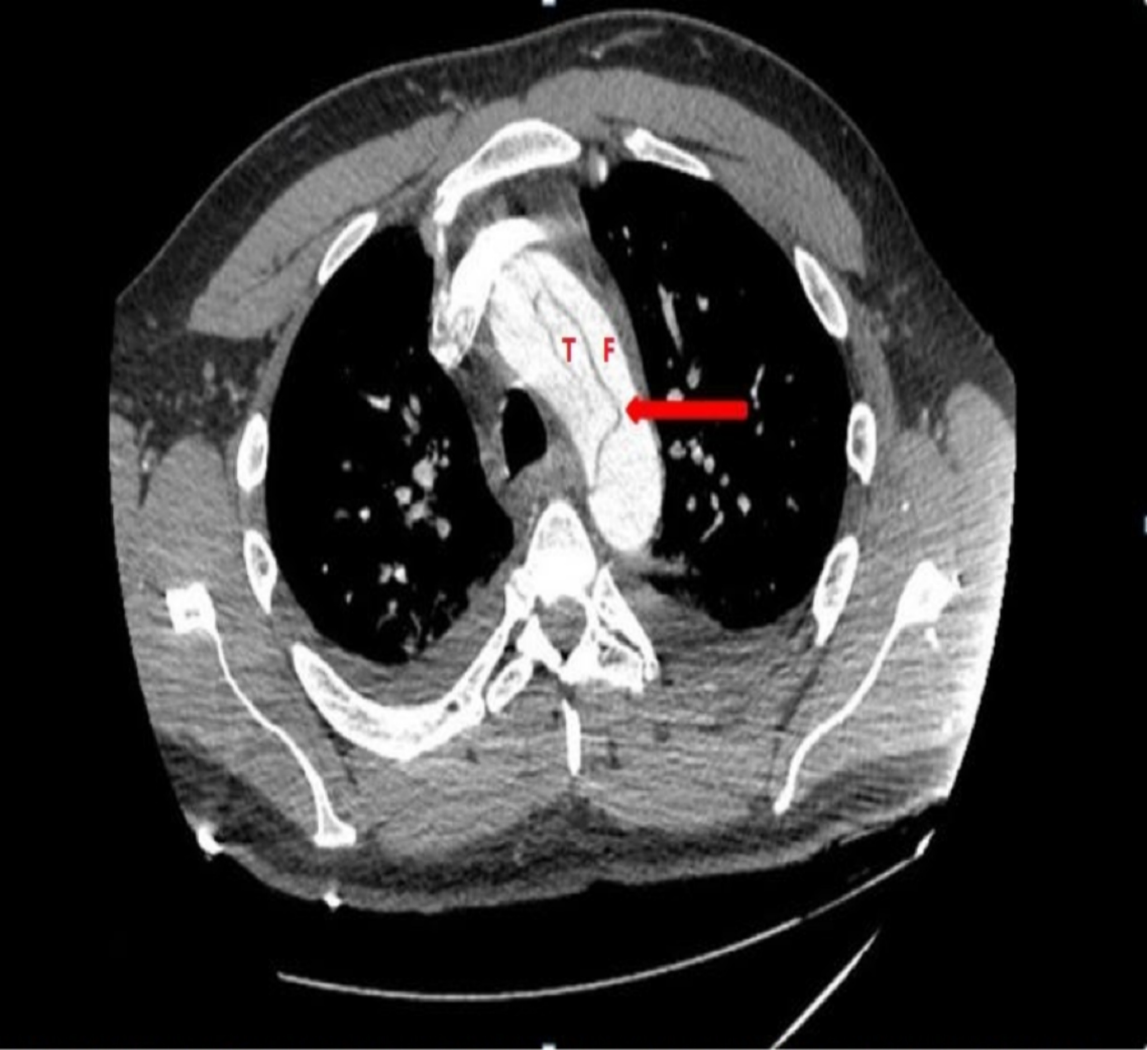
Computed tomography showing aortic dissection with arrows pointing towards the intimal tear in the aortic arch separating the true lumen (T) from the false lumen (F).
